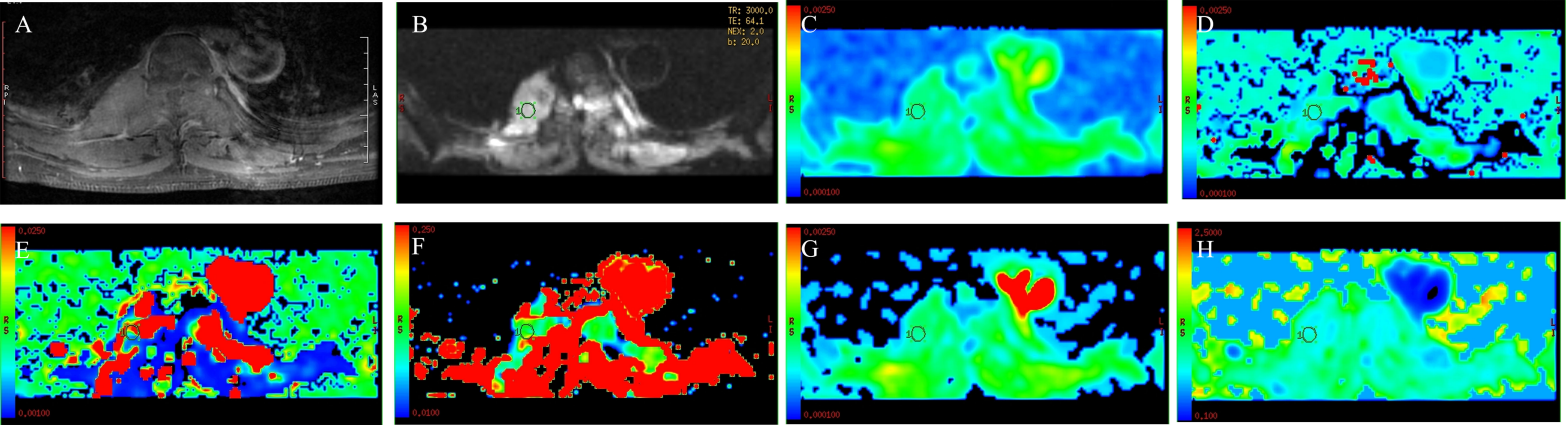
A 44-year-old woman with spinal tuberculosis. The lesion showed obvious enhancement on T1WI (A) and hyperintensity on DWI (B). ADC values were 1.28 × 10^−3^ mm^2^/s (C). The ROI was placed on DWI (B) and copied to the other IVIM parametric maps of ADC_slow_, ADC_fast_, f, DDC, and α (D–H) with values of 1.11 × 10^−3^ mm^2^/s, 0.0131 mm^2^/s, 0.190, 1.41 × 10^−3^ mm^2^/s, and 0.807, respectively.
